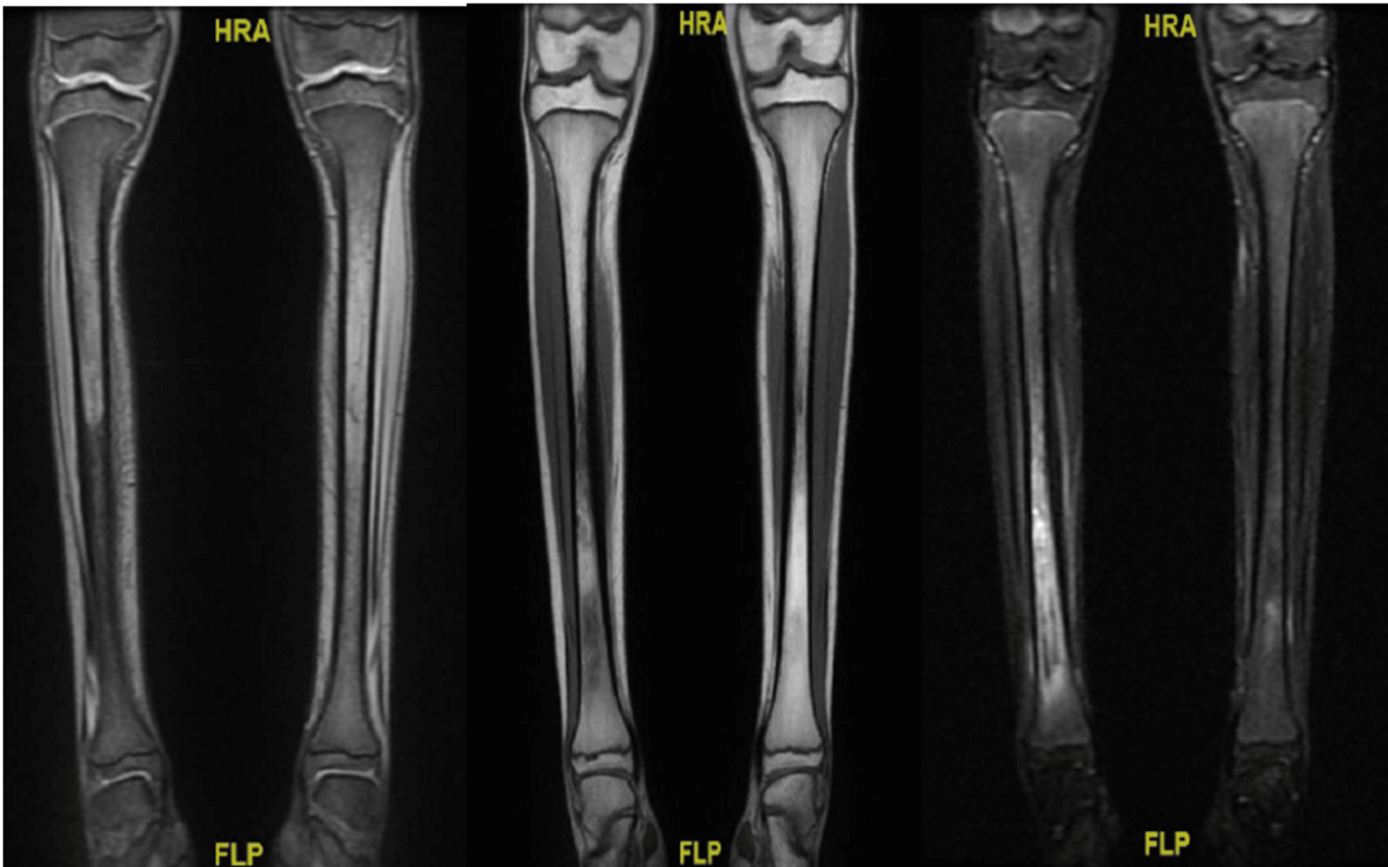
MRI of the Right Tibial Shaft Showing Diffuse Altered Bone Marrow Signal With Soft Tissue Changes MRI of the right tibial shaft shows a diffuse altered bone marrow signal, characterized by heterogeneous low T1 and bright T2/short tau inversion recovery (STIR) signals with enhancement. Cortical thickening and mild edematous changes in the surrounding soft tissue are also noted. HRA: high-resolution anatomical; FLP: finger-like projection
